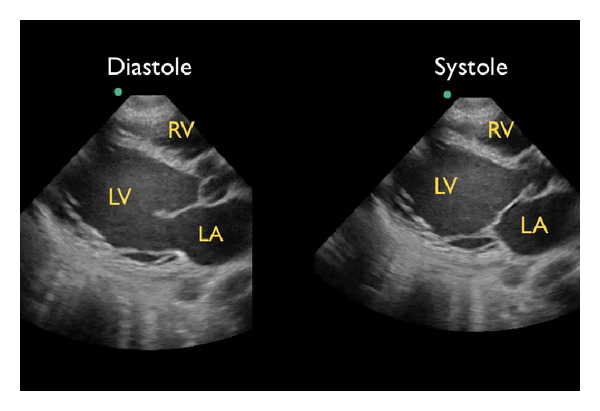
Poor left ventricular contractility, parasternal long axis view. RV: right ventricle, LV: left ventricle, LA: left atrium.
